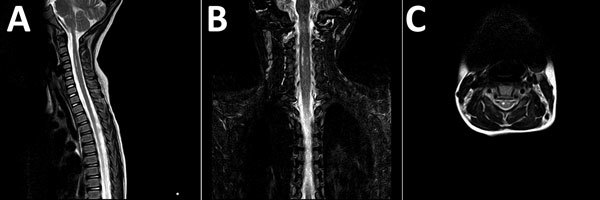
Magnetic resonance images of brain of a 3-year-old girl with enterovirus encephalomyelitis (paresis of the right upper limb). A) Image of the cervical spine: sagittal T2 sequence; B) short tau inversion recovery (STIR) coronal sequence; C) T2 axial sequence. Hyperintense filiform lesions in the anterolateral regions of the spinal cord (C3–C5), predominantly right, are suggestive of myelitis.
